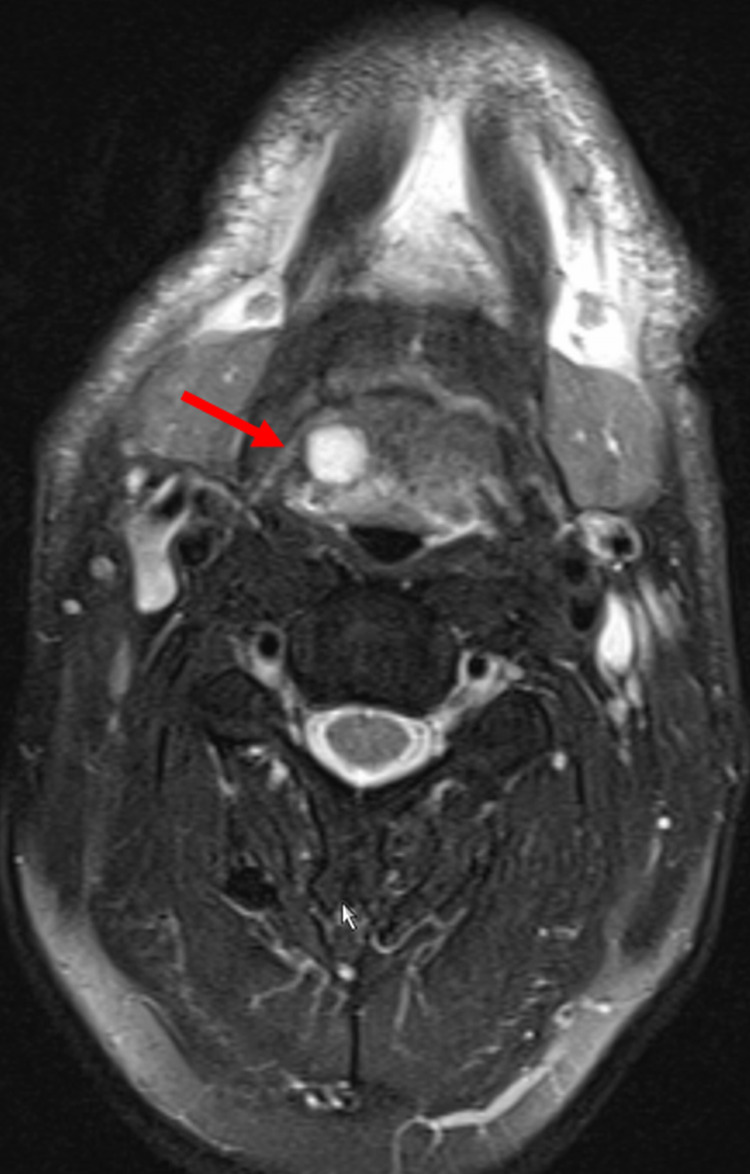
Tongue root cyst in a m.3243A>G carrier Axial, T1-weighted MRI image of the collum showing a hyperintense lesion in the right tongue root, interpreted as thin- and smooth-walled, pre-epiglottic cyst with 1 cm in diameter and protein-rich content, which did not enhance after application of gadolinium.
